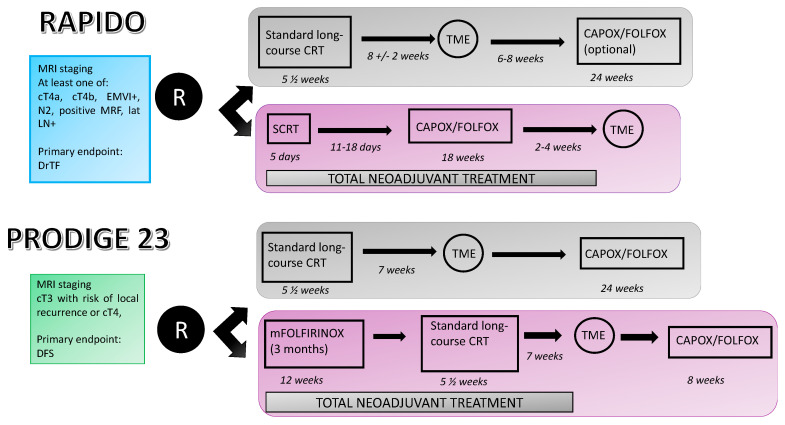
Comparison of RAPIDO and PRODIGE 23 trial designs. MRI: magnetic resonance imaging; EMVI: extra-mural vascular invasion; lat LN+: lateral lymph nodes involved; DrTF: disease-related treatment failure; MRF: mesorectal fascia; DFS: disease-free survival; CRT: chemoradiotherapy; TME: total mesorectal excision; SCRT: short-course radiotherapy.
